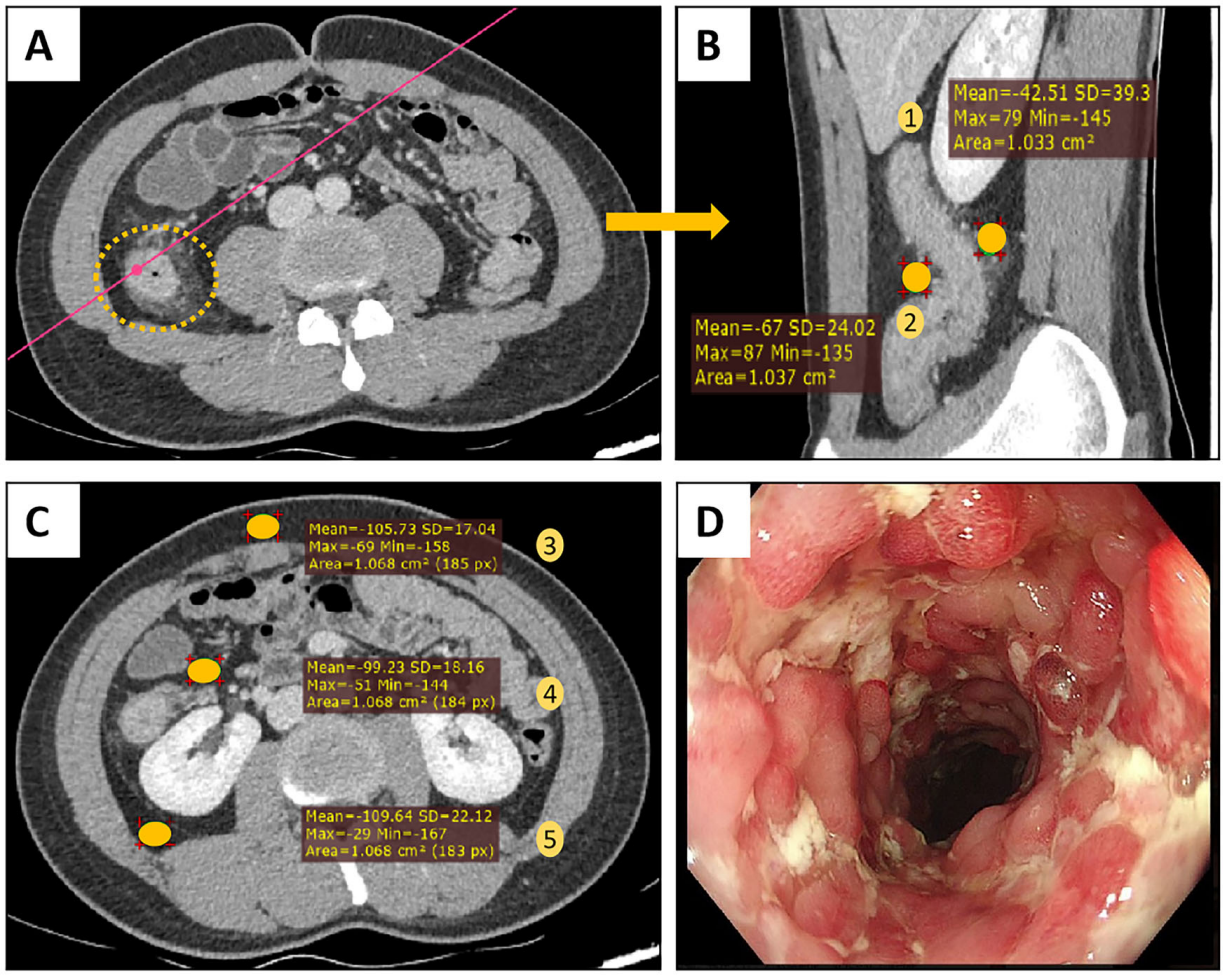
Quantitative assessment of the fat attenuation index (FAI) at 5 different locations. Abdominal CT image of a 22-year-old Crohn’s disease patient whose most severe bowel lesion was located at the ascending colon. A The yellow circle indicated the bowel lesion. B The oblique sagittal section is reconstructed based on the pink line. C Transverse section of the third lumbar vertebra (L3). D Endoscopic image of the bowel lesion. The numbers 1–5 represent 5 different locations, namely, the mesenteric side (MS) and the opposite side of MS (OMS) around the most severe bowel lesion, the subcutaneous area, the space around the normal bowel wall (Nor) and the retroperitoneal space (RS), respectively
